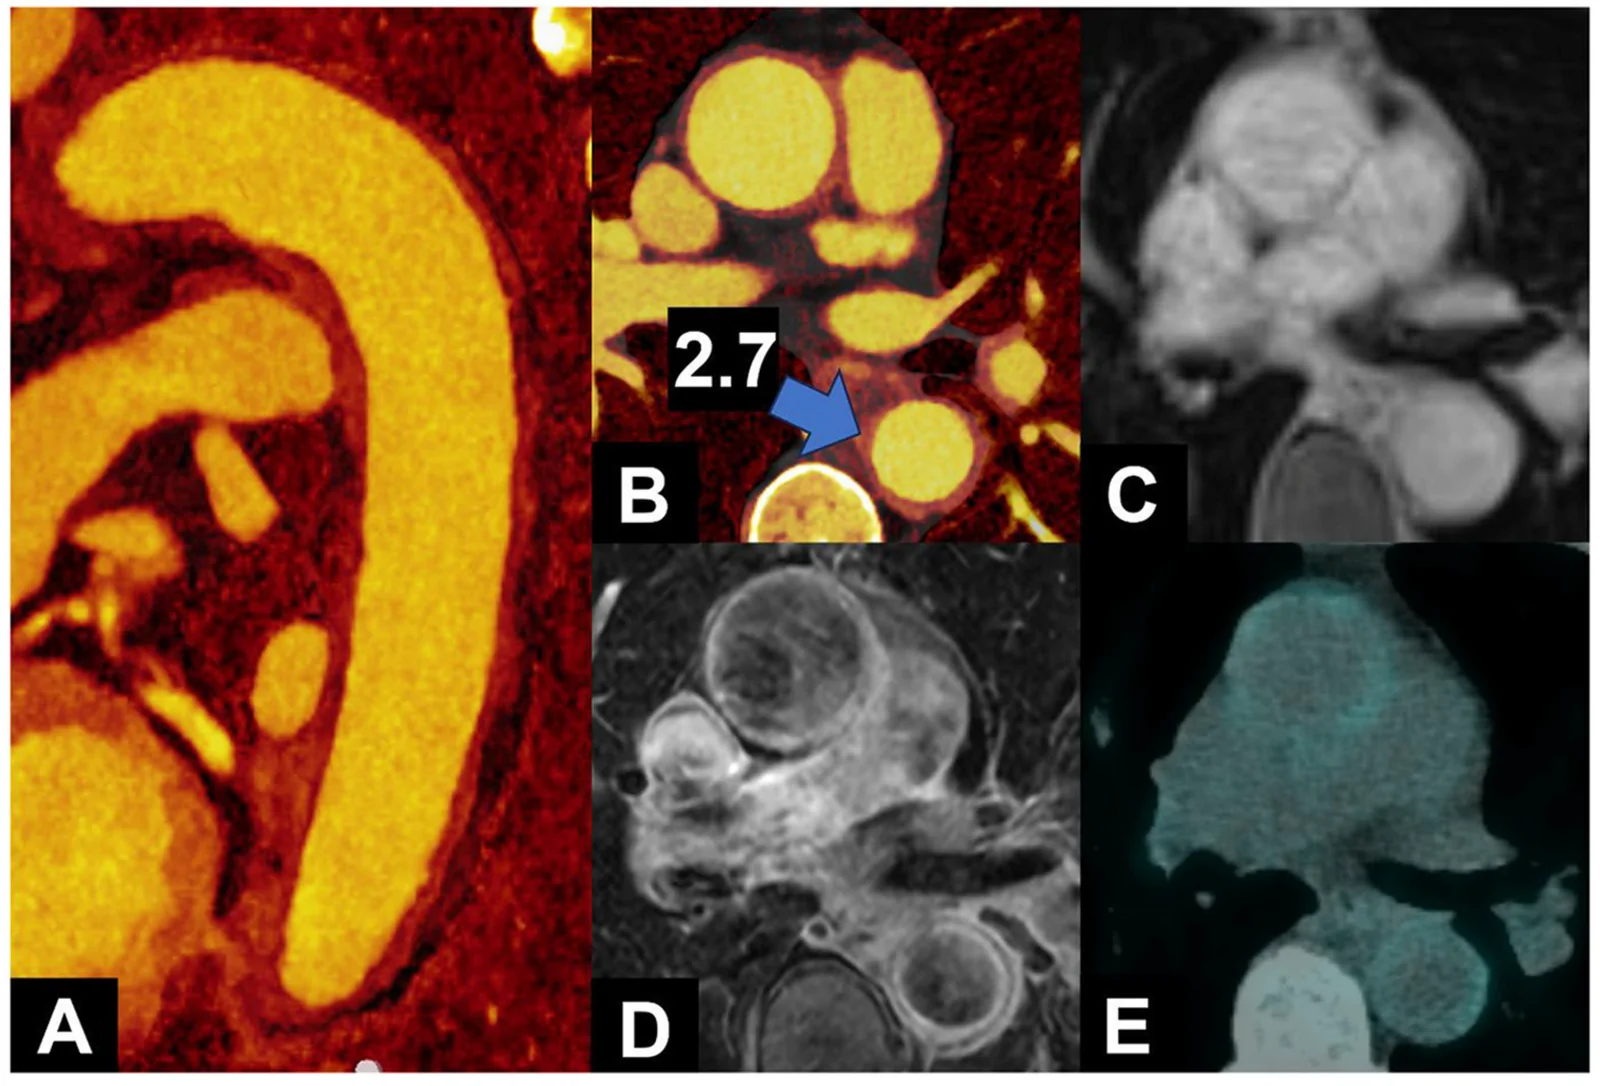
PCCT evaluation of the thoracic aorta in a 69-year-old woman during the venous phase. (A,B) Color-coded iodine maps show circumferential aortic wall thickening with iodine uptake of 2.7 mg I/mL. (C,D) T1-weighted late-enhancement and dark-blood MRI confirm the wall thickening and increased gadolinium enhancement. (E) Subsequent 18F-FDG PET/CT demonstrates increased tracer uptake, supporting the diagnosis of large-vessel vasculitis [103].
